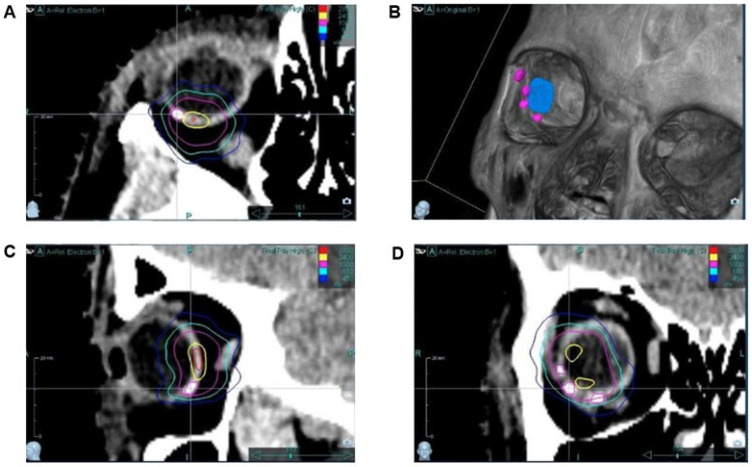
CT images of the silicone markers ((A) transverse; (C) sagittal; (D) coronal). Image (B) is the result of the VRT (volume rendering technique). Three of the markers were directly placed at the peripheral edges of the tumor, whereas the fourth marker was placed contralaterally. Tumor size (blue) was defined via fundus image, OCT and ultrasonography. In detail, tumor diameters and the distance of the tumor from the optic nerve and the macula measured with the 3 examination techniques were used to determine the tumor boundaries far from the markers. All markers could be clearly detected without scattering artifacts.
